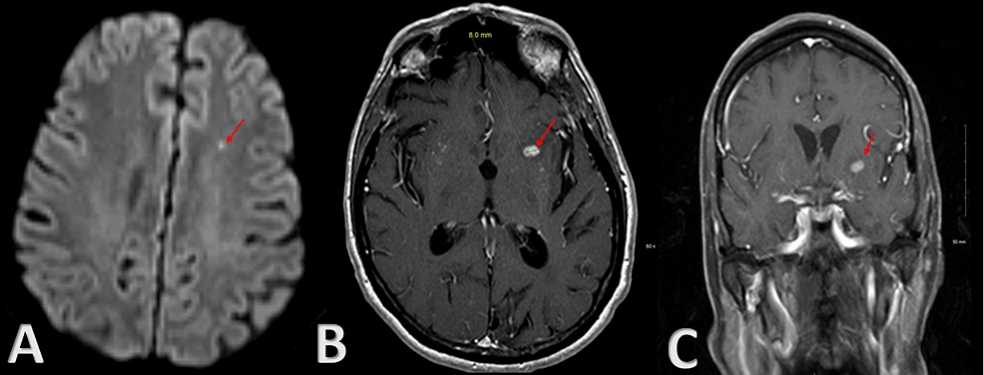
(A) Axial DWI sequence MRI brain demonstrating small acute ischemic infarct in the left precentral gyrus as well as a 2 cm mass lesion in the left basal ganglia (red arrow). (B) Axial T1 sequence MRI brain with contrast demonstrating a 2 cm mass lesion in the left basal ganglia (red arrow). (C) Coronal T1 sequence MRI brain with contrast demonstrating a 2 cm mass lesion in the left basal ganglia (red arrow). DWI: diffusion-weighted imaging; MRI: magnetic resonance imaging
